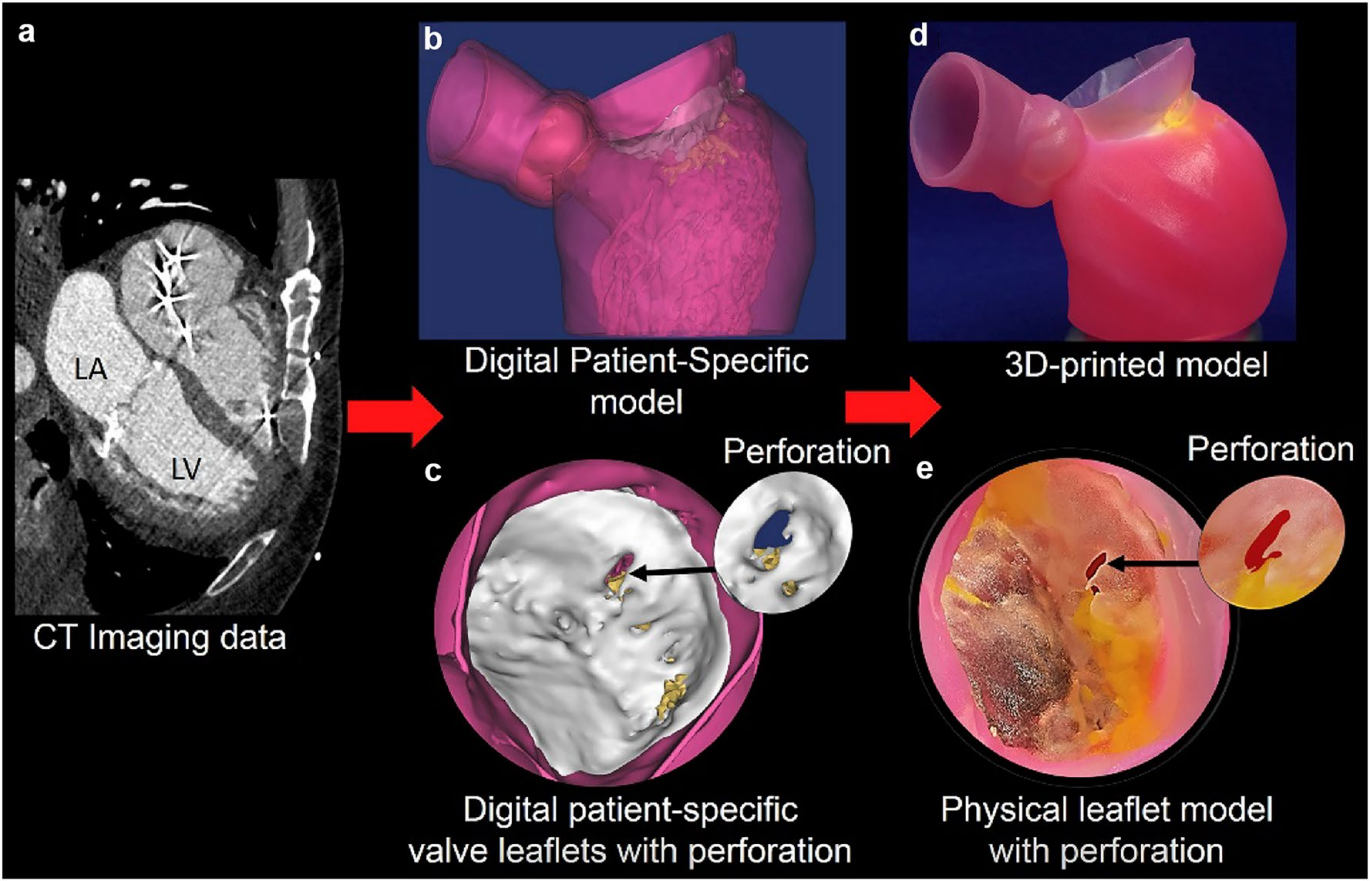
3D printing of Mitral Value geometry with regional calcium deposition (yellow) and Pathology shown in model E in the above figure. a CT Image b Digital patient-specific model c Digital patient-specific valve leaflets with perforation d 3D printed model e Physical leaflet model with perforation [84]
